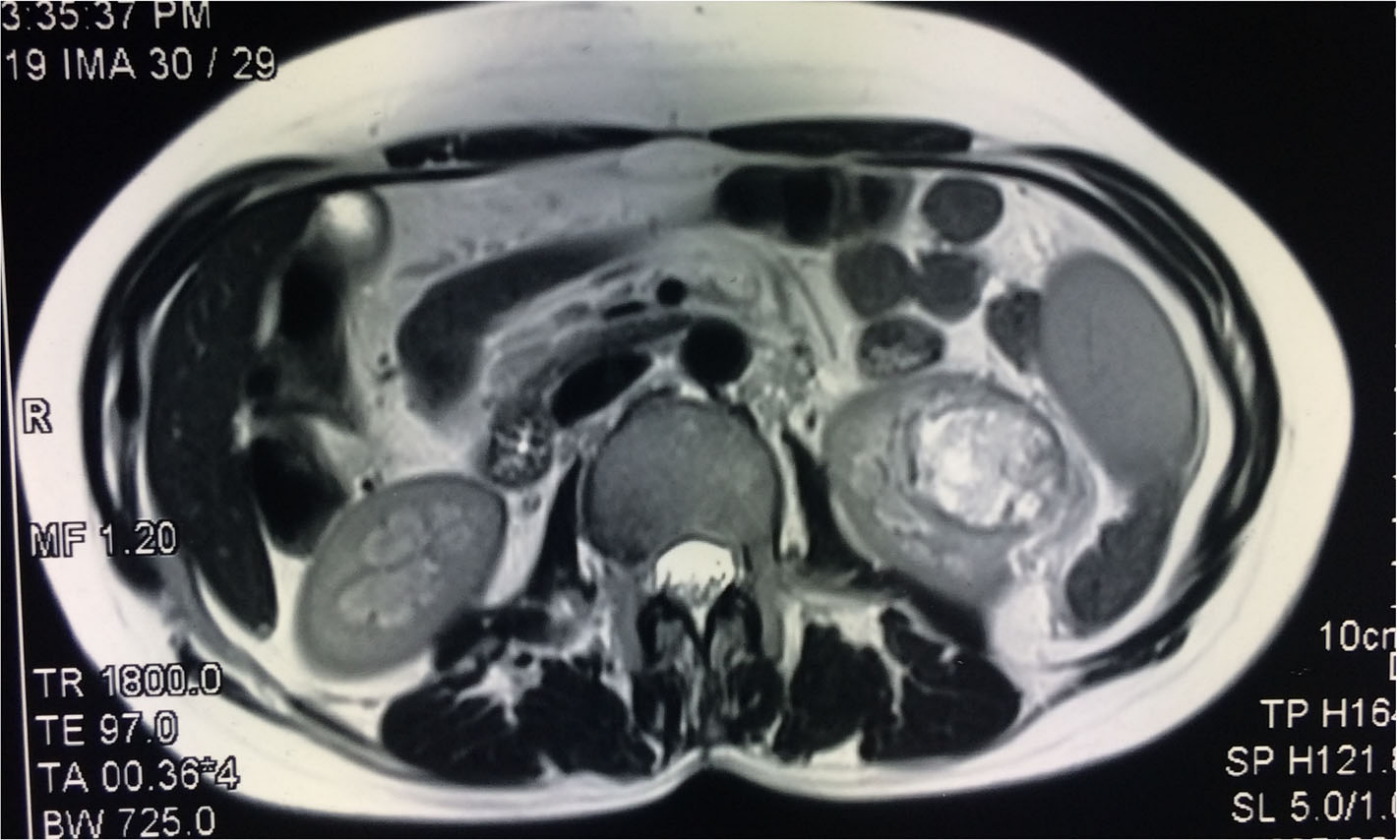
MRI (transverse section) showing enhanced renal mass at lower pole of left kidney
